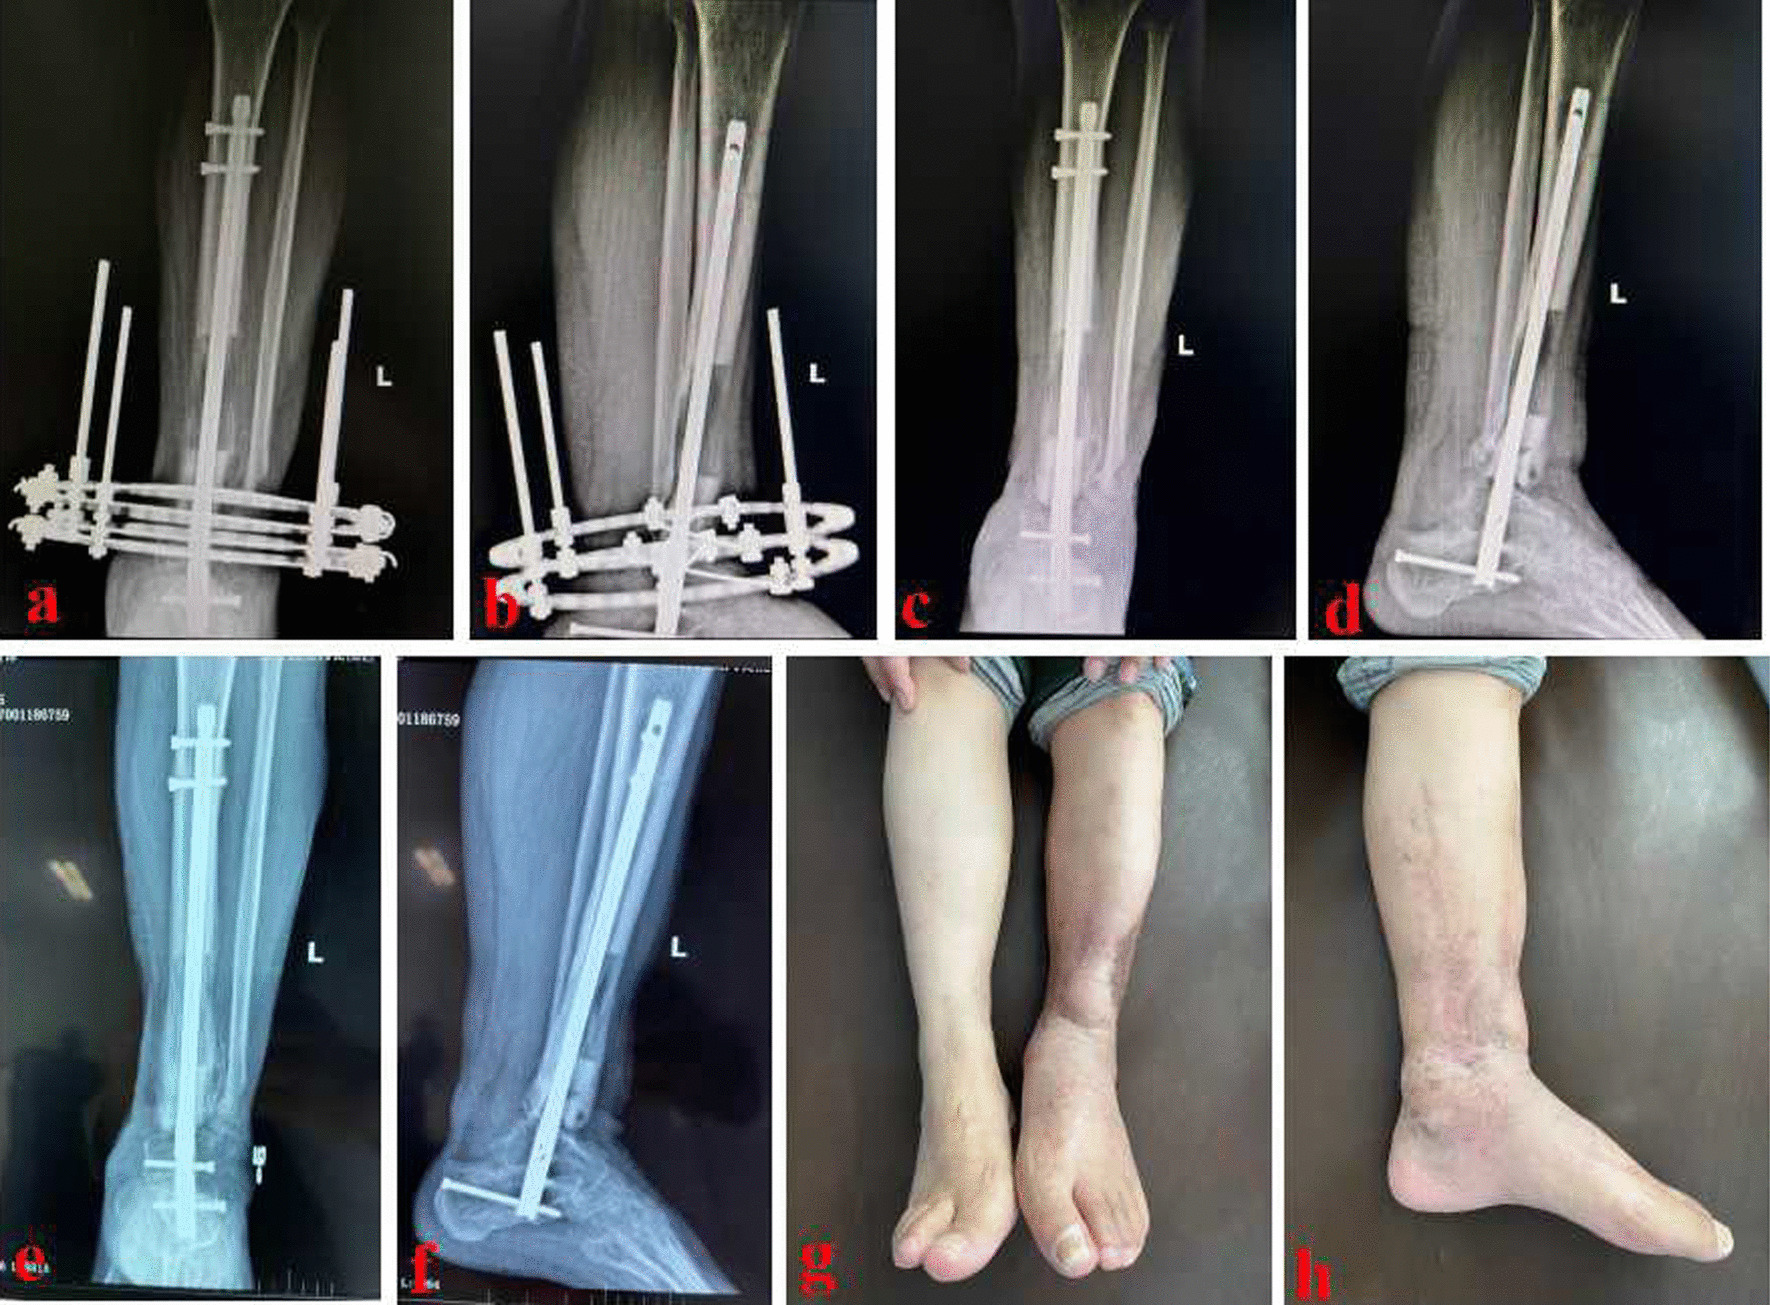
The patient underwent bone transport and was successfully treated using the MHT technique. a and b After three months, the bone transport period finished and new callus was generated; c and d X-ray films after removing the annular transport frame; e and f After six months, X-ray films showed an appropriate consolidation of new callus; g and h Appearance after the reconstruction of distal tibial defects. MHT: modified hybrid transport
